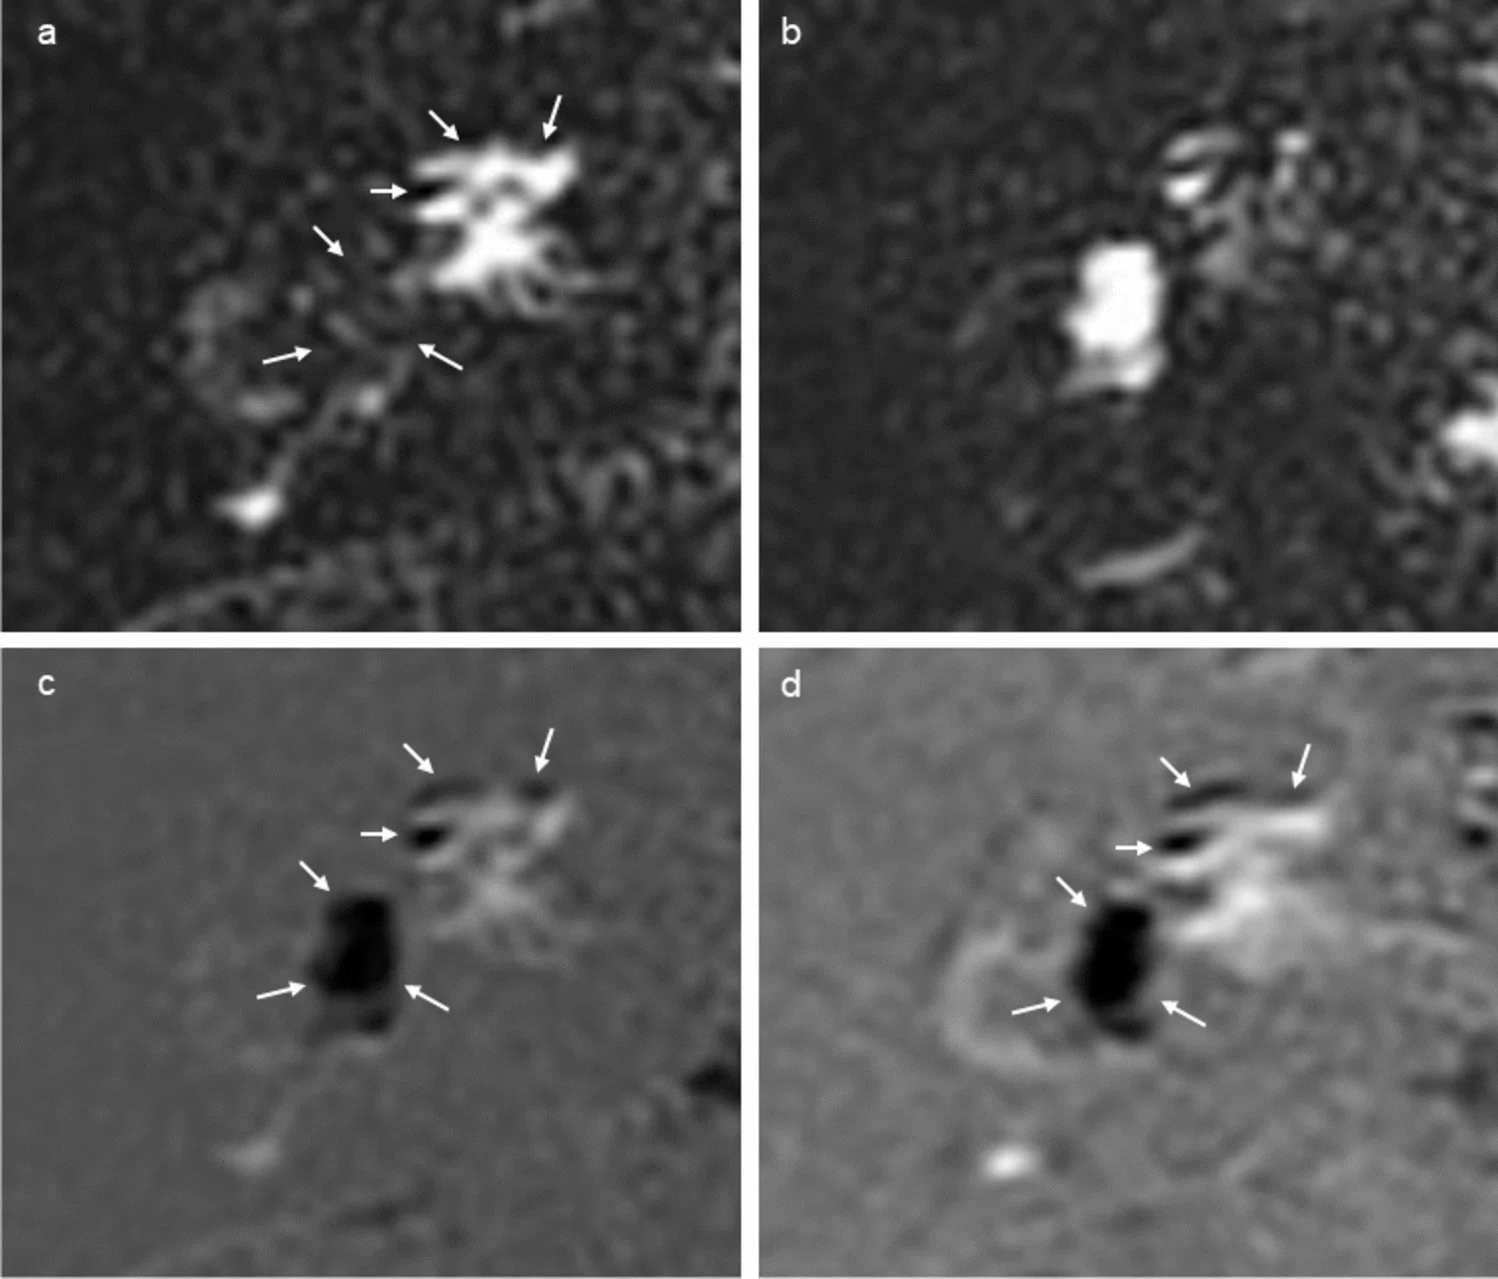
A woman with Meniere’s disease in her 30 s. Images are obtained 4 h after intravenous administration of single dose GBCA. a Heavily T2-weighted 3D-FLAIR image; the positive perilymph image (PPI, TR/TE/TI:16,000/544/2900). Perilymph shows bright signals by the distribution of GBCA. b The positive endolymph image (PEI, TR/TE/TI: 16,000/544/2500). Endolymph without the distribution of GBCA shows bright signals. c HYDROPS (HYbriD of reversed image of positive endolymph signal and native image of positive perilymph signal) is generated by the subtraction of PEI from PPI.Separation between bright perilymph, black endolymph, and gray bone is possible on a single image. Significant endolymphatic hydrops is observed in the cochlea and the vestibule (arrows). d The 3D-real IR, PRISM (TR/TE/TI: 16,000/538/2700). Endolymphatic hydrops in the cochlea and the vestibule (arrows) are visualized almost similarly as HYDROPS image. Note that the boundary between the endolymph (short arrows) and the adjacent bone and air is indistinct on the 3D-FLAIR image (a), whereas it is clearly delineated on the HYDROPS (c) and PRISM (d) images. The images are reprinted from the reference under the Creative Commons Attribution-NonCommercial-NoDerivatives International License [16]. GBCA, gadolinium-based contrast agent; PRISM, Polarity-preserving Real Inversion Solute Mapping
